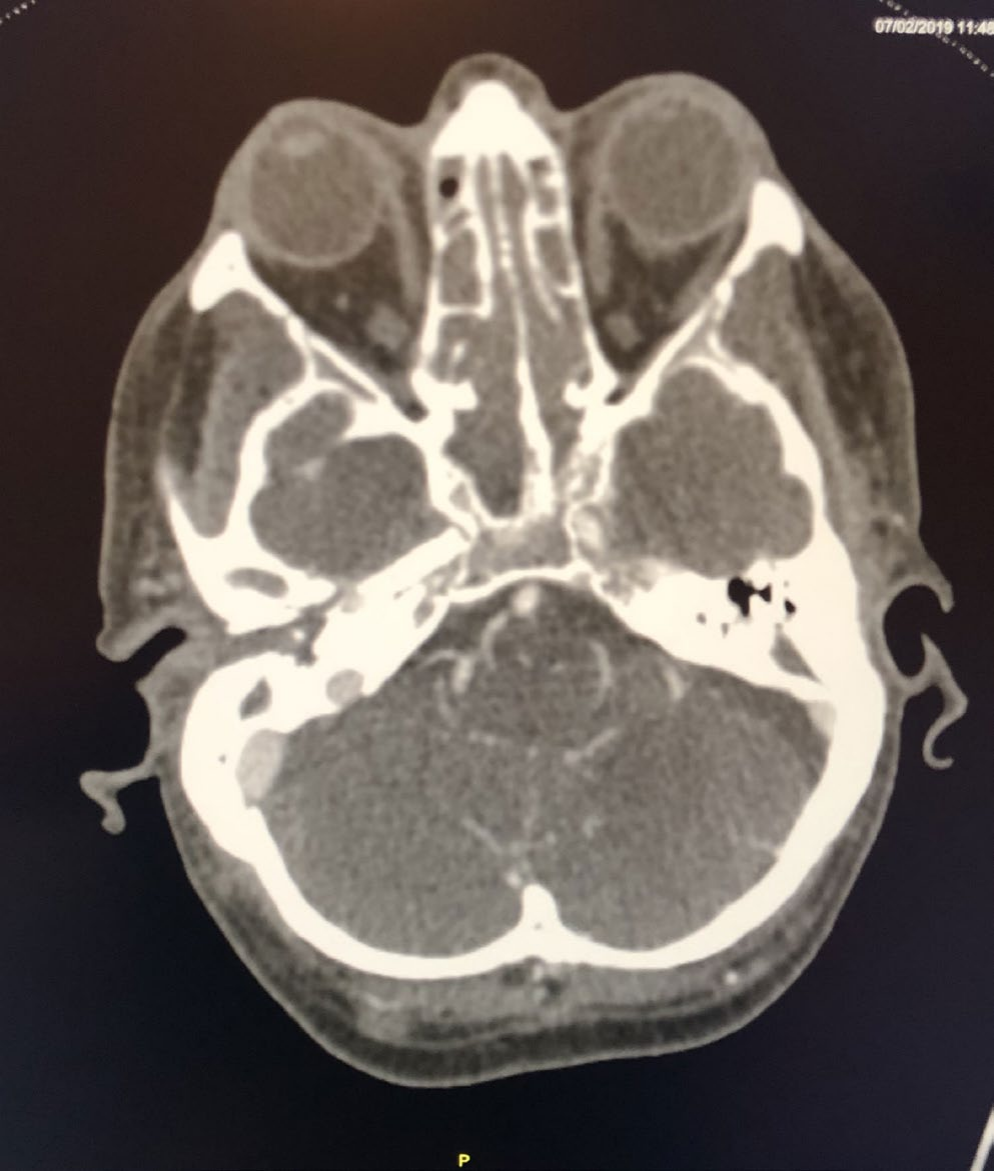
CT angiogram post stent placement
